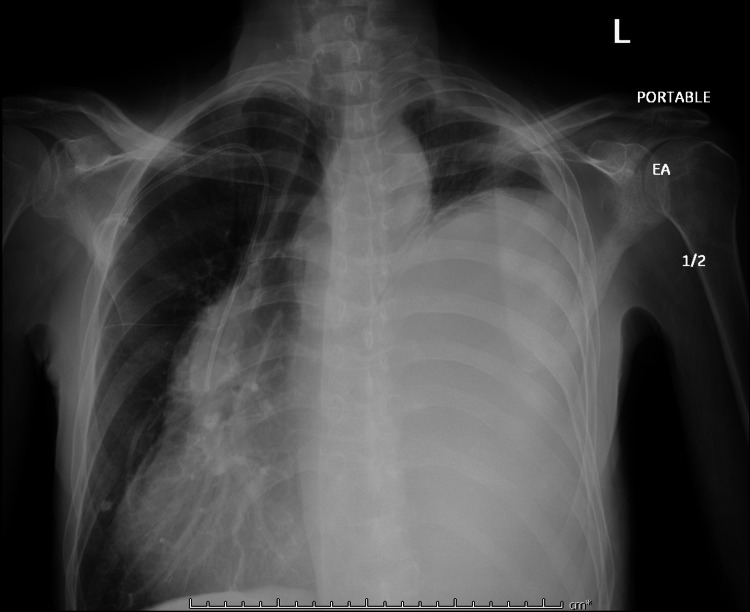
Chest X-ray on admission now showing increase in left-sided pleural effusion
